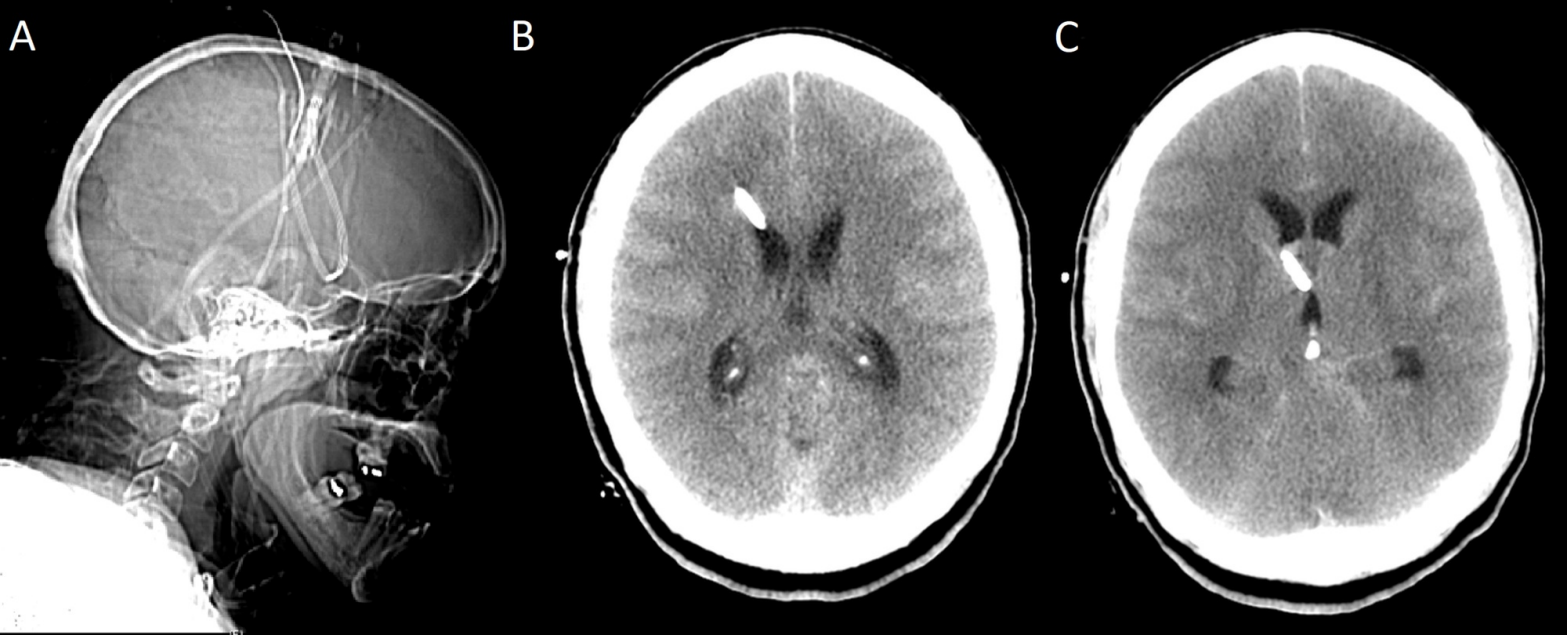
Sagittal scout image (A), Axial initial CT head scans following external ventricular drains (EVD) insertion (B and C) showing catheter tip terminating at Foramen of Monro
